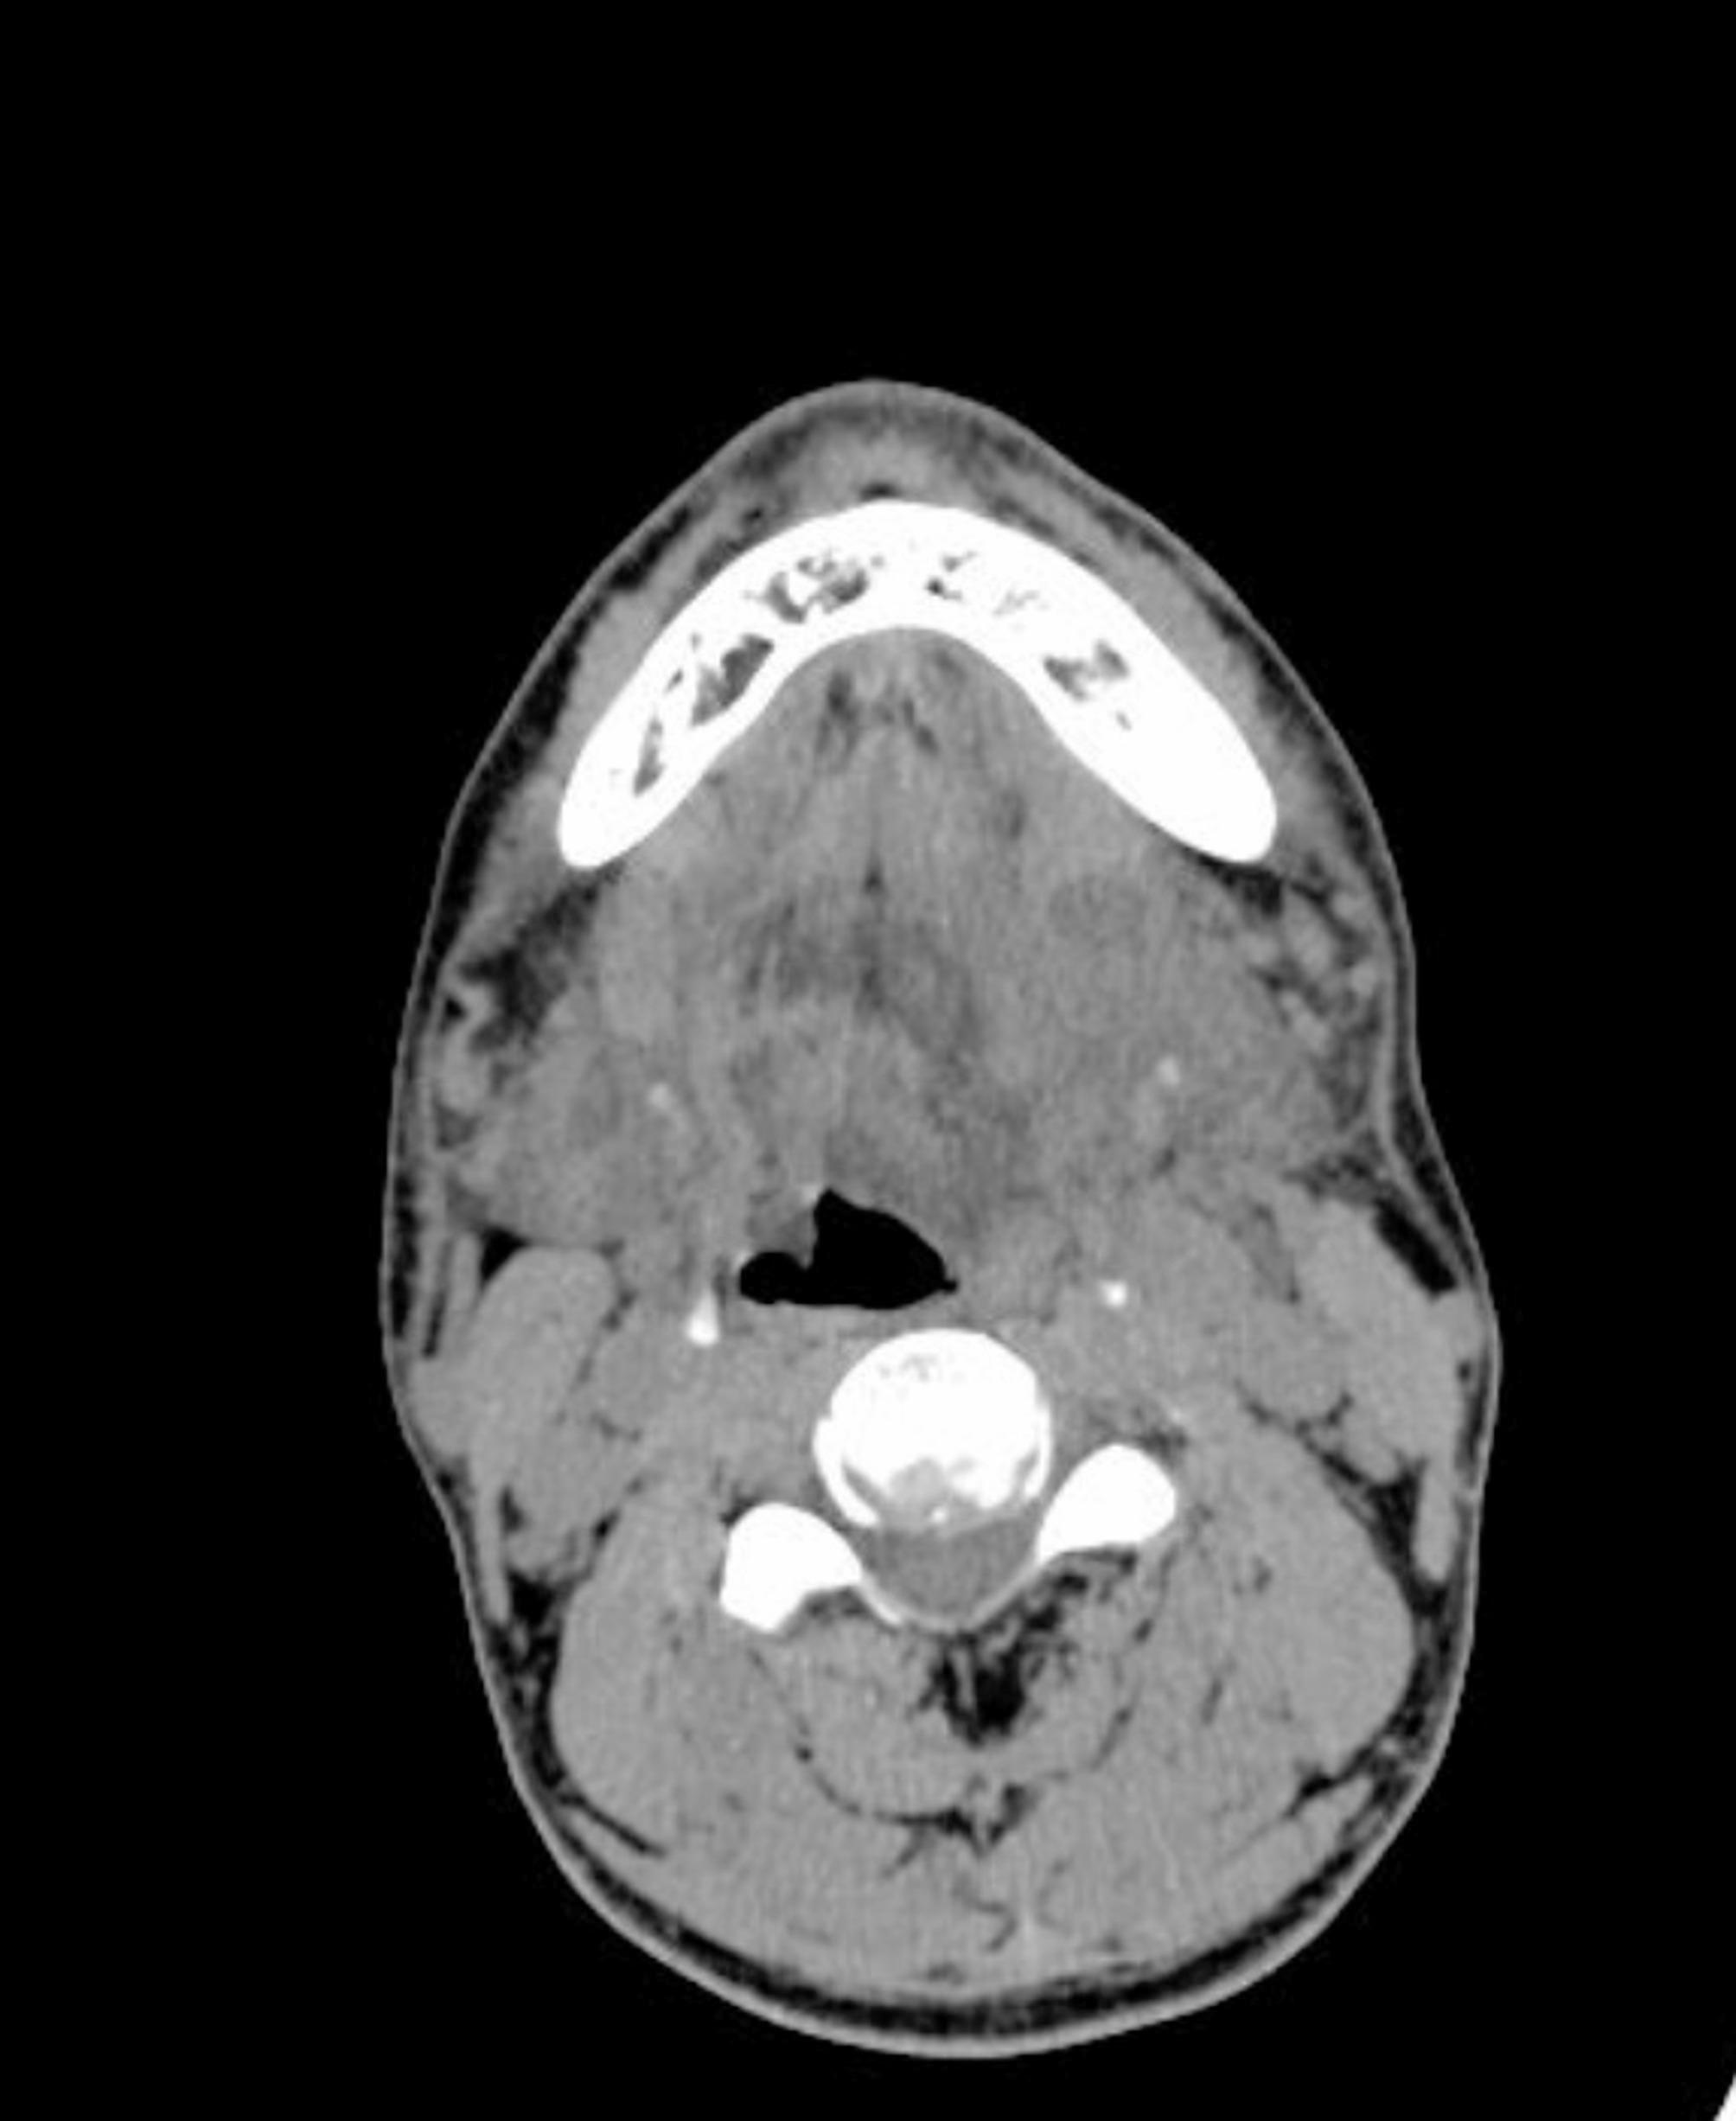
Axial CT at the level of the neck revealed diffuse swelling in the floor of the mouth and the submandibular regions
